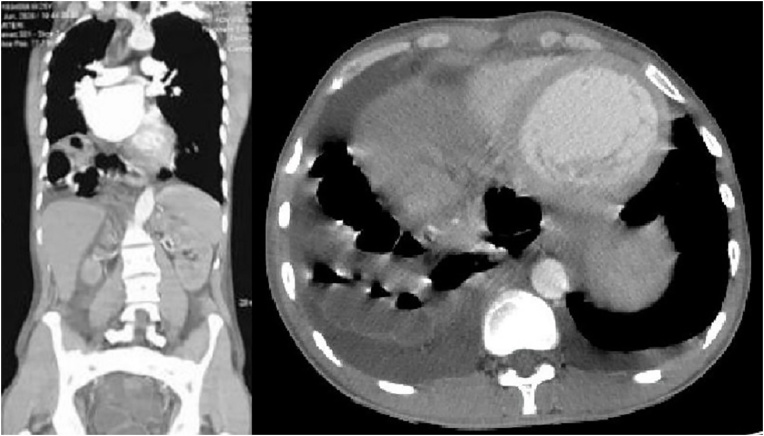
Abdominal CT-Scan showing colonic loop present between the right hemidiapraghma and liver.
